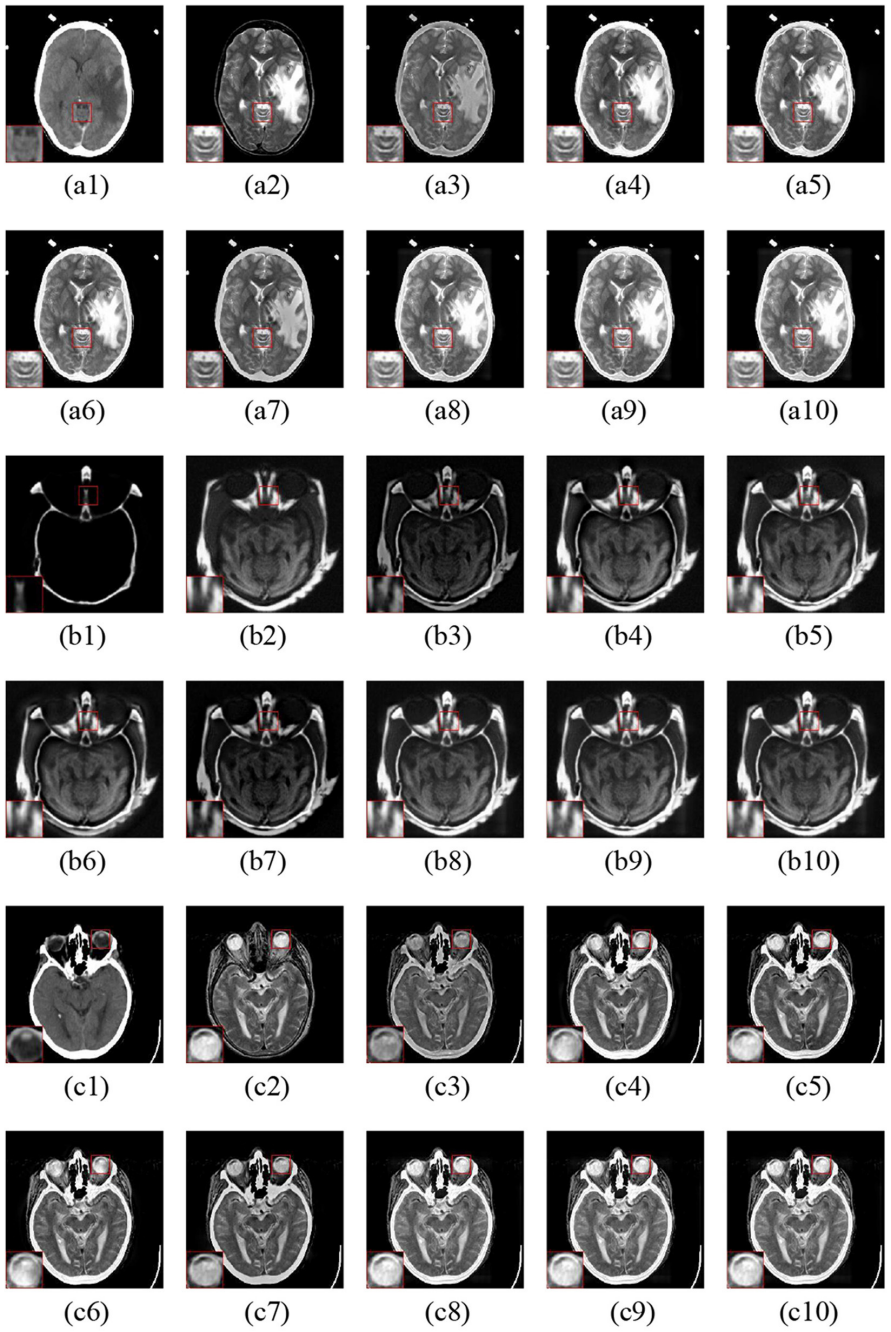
Three fusion examples of CT and MR images. One close-up is provided in each set for better comparison. The original images: (a1–c1) CT and (a2–c2) MR. The fusion results (a3–c3) ASR, (a4–c4) NSCT-PC-LLE, (a5–c5) LP-CNN, (a6–c6) NSST-PAPCNN, (a7–c7) CSMCA, (a8–c8) LatLRR-FCN-32s, (a9–c9) LatLRR-FCN-16s, and (a10–c10) LatLRR-FCN-8s.
